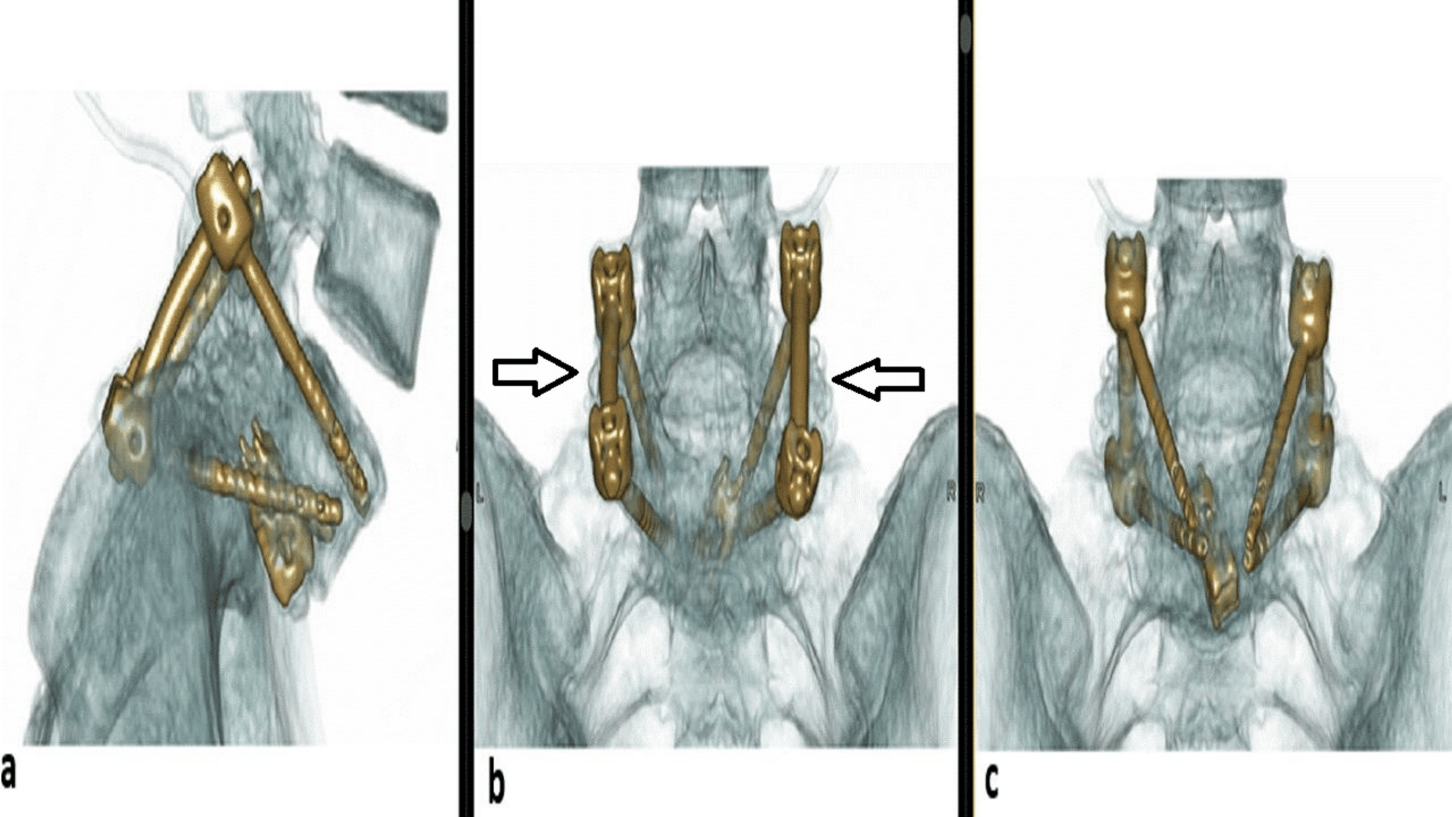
3D CT scan reconstruction showing the positioning of the screws and expandable cage six months postoperatively with solid fusion posterolateral (arrows): (a) lateral, (b) posterior, and (c) anterior view.
